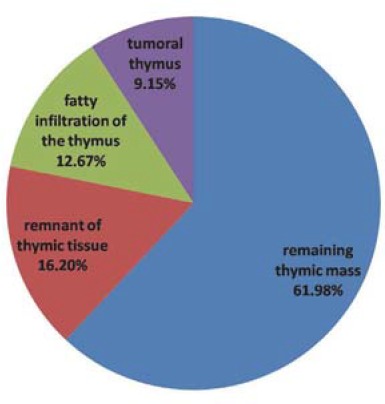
CT aspects in all patients with thymic lymphoid follicular hyperplasia
